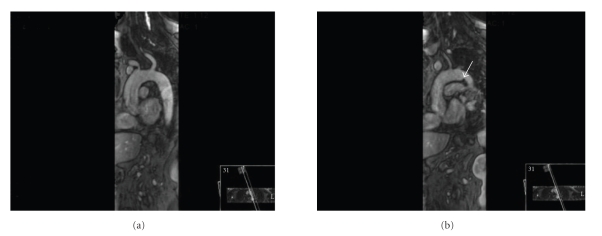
Thoracic MRI performed before surgery, showing normal dimensions of the thoracic aorta (a) and a repletion defect (*arrow*) in the aortic arch-descending thoracic aorta transition compatible with a vegetation (b).
